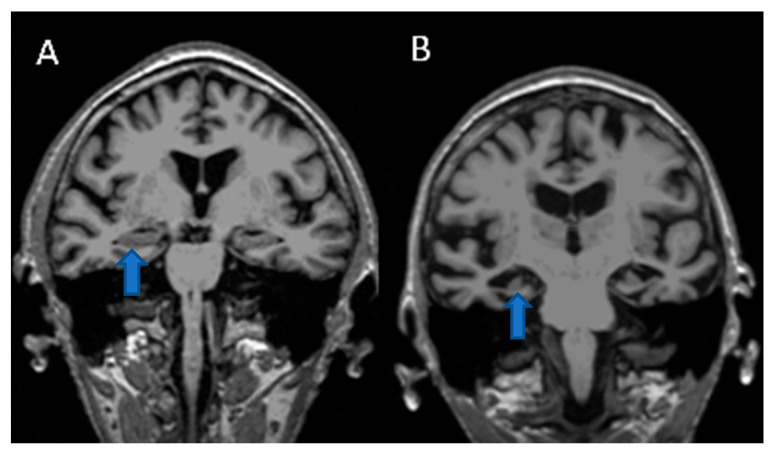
MRI brain images (blue arrows point to hippocampus) from (A) a control patient and (B) an Alzheimer’s disease patient.
